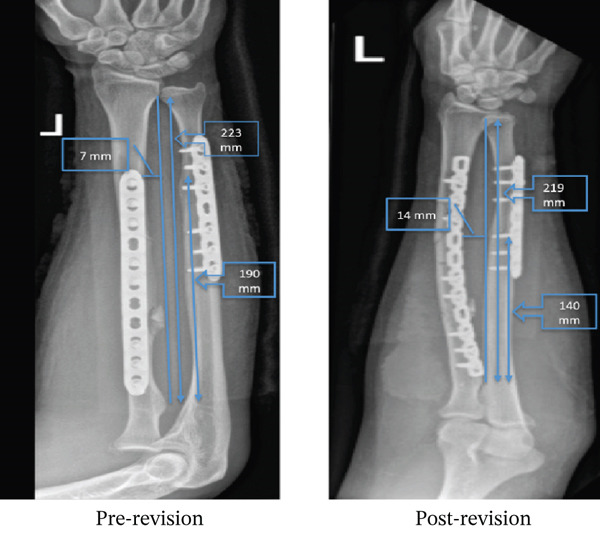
Prerevision and postrevision radiographs and measurements of maximum radial bow and location of maximum radial bow.
